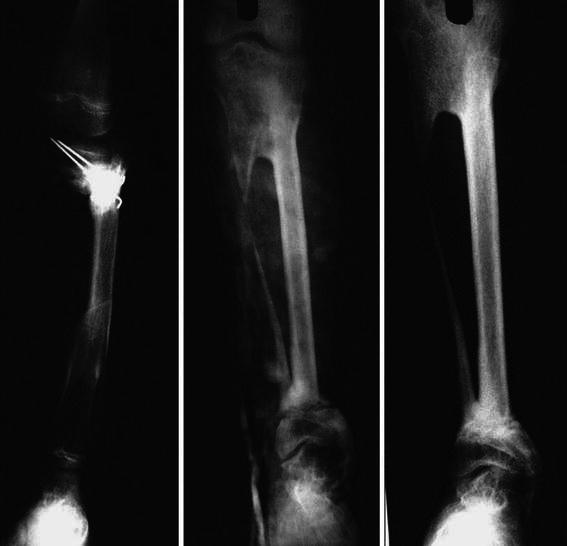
Radiograph showing additional procedures for the correction of the proximal tibial varus deformity at the knee and marked varus deformity at the ankle joint in two different patients
